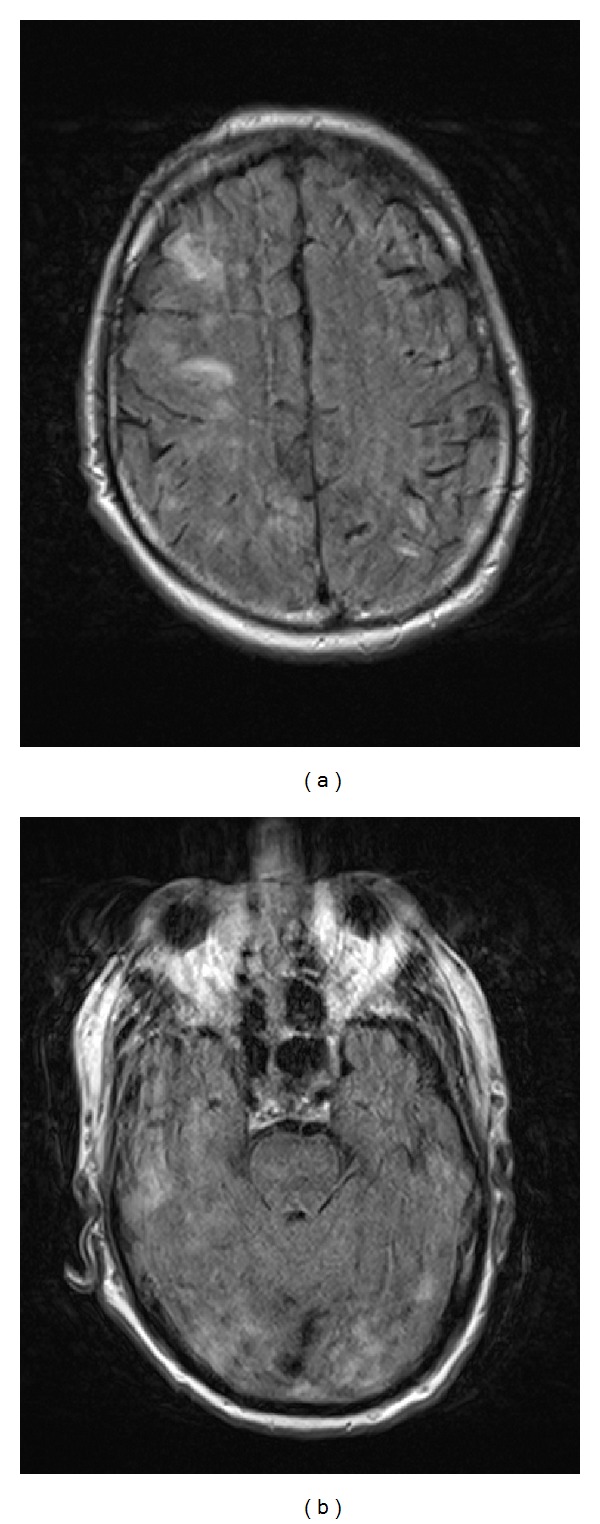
FLAIR AXIAL MRI images (TR 9000, TE 112) from the second admission demonstrating significant interval worsening of bilateral cortical hyperintensities throughout both cerebral hemispheres. Note the mild patient motion artifact.
